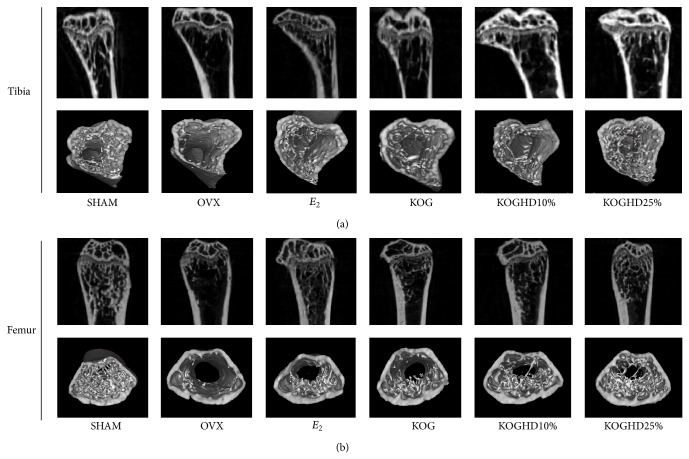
Analysis of microcomputed tomography in the region of the proximal tibia and distal femur after sacrifice. Representative 3D images of (a) tibia and (b) femur.
